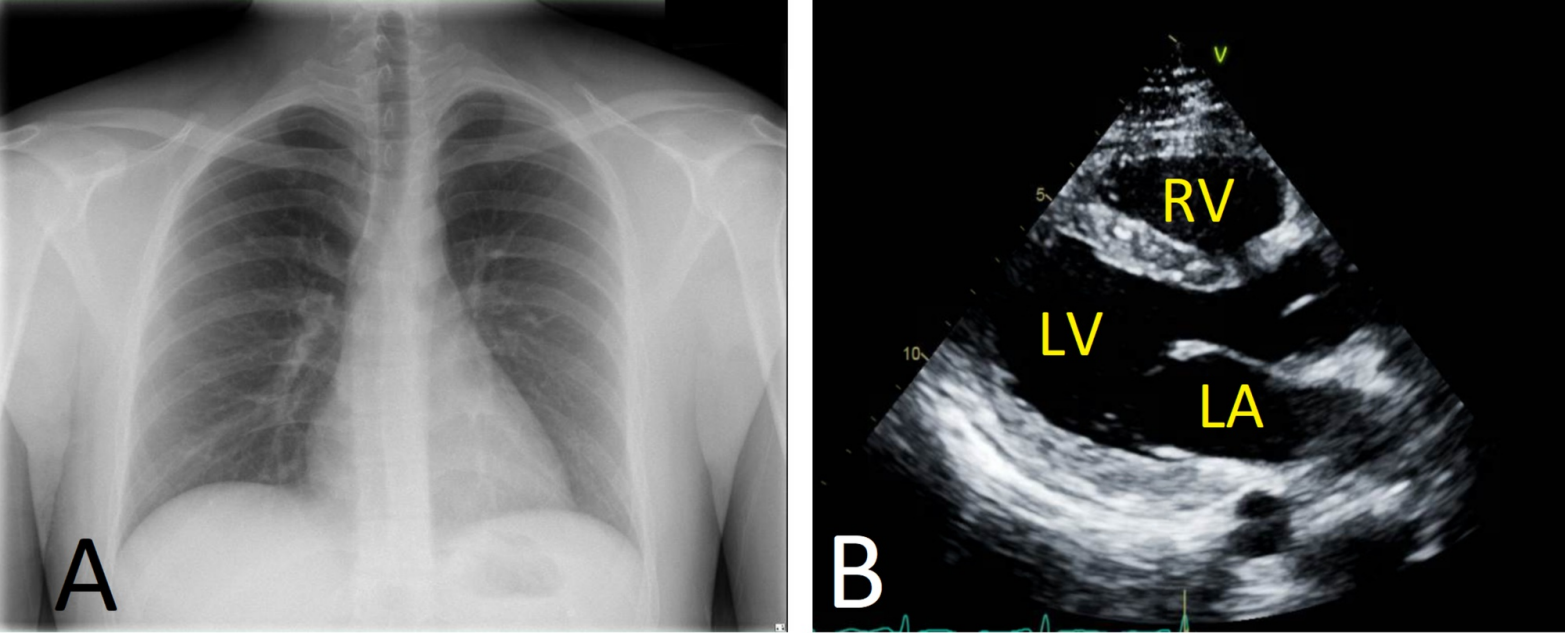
Imaging upon follow-up post pericardiocentesis. (A) Chest X-ray showing the normal size of the heart. (B) Transthoracic echocardiography: parasternal long axis view demonstrating resolution of pericardial effusion. LV: left ventricle; LA: left atrium; RV: right ventricle.
